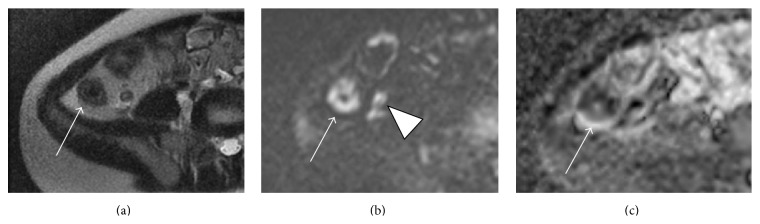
Diffusion-weighted imaging of active Crohn's disease. (a) Axial single-shot T2-weighted image demonstrating wall thickening and edema in the terminal ileum; (b) DWI and (c) ADC images (*b* = 600) demonstrating hyperintense signal on DWI and low ADC (arrow) in the same region of the terminal ileum indicating restriction of diffusion. There is also restricted diffusion in the distal ileum and appendix (arrowheads) consistent with additional areas of inflammation.
